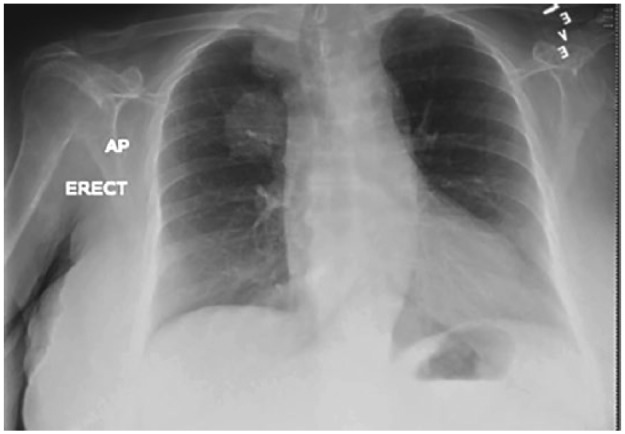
Chest X-ray showing a 4.3 cm right upper lobe lung mass.
